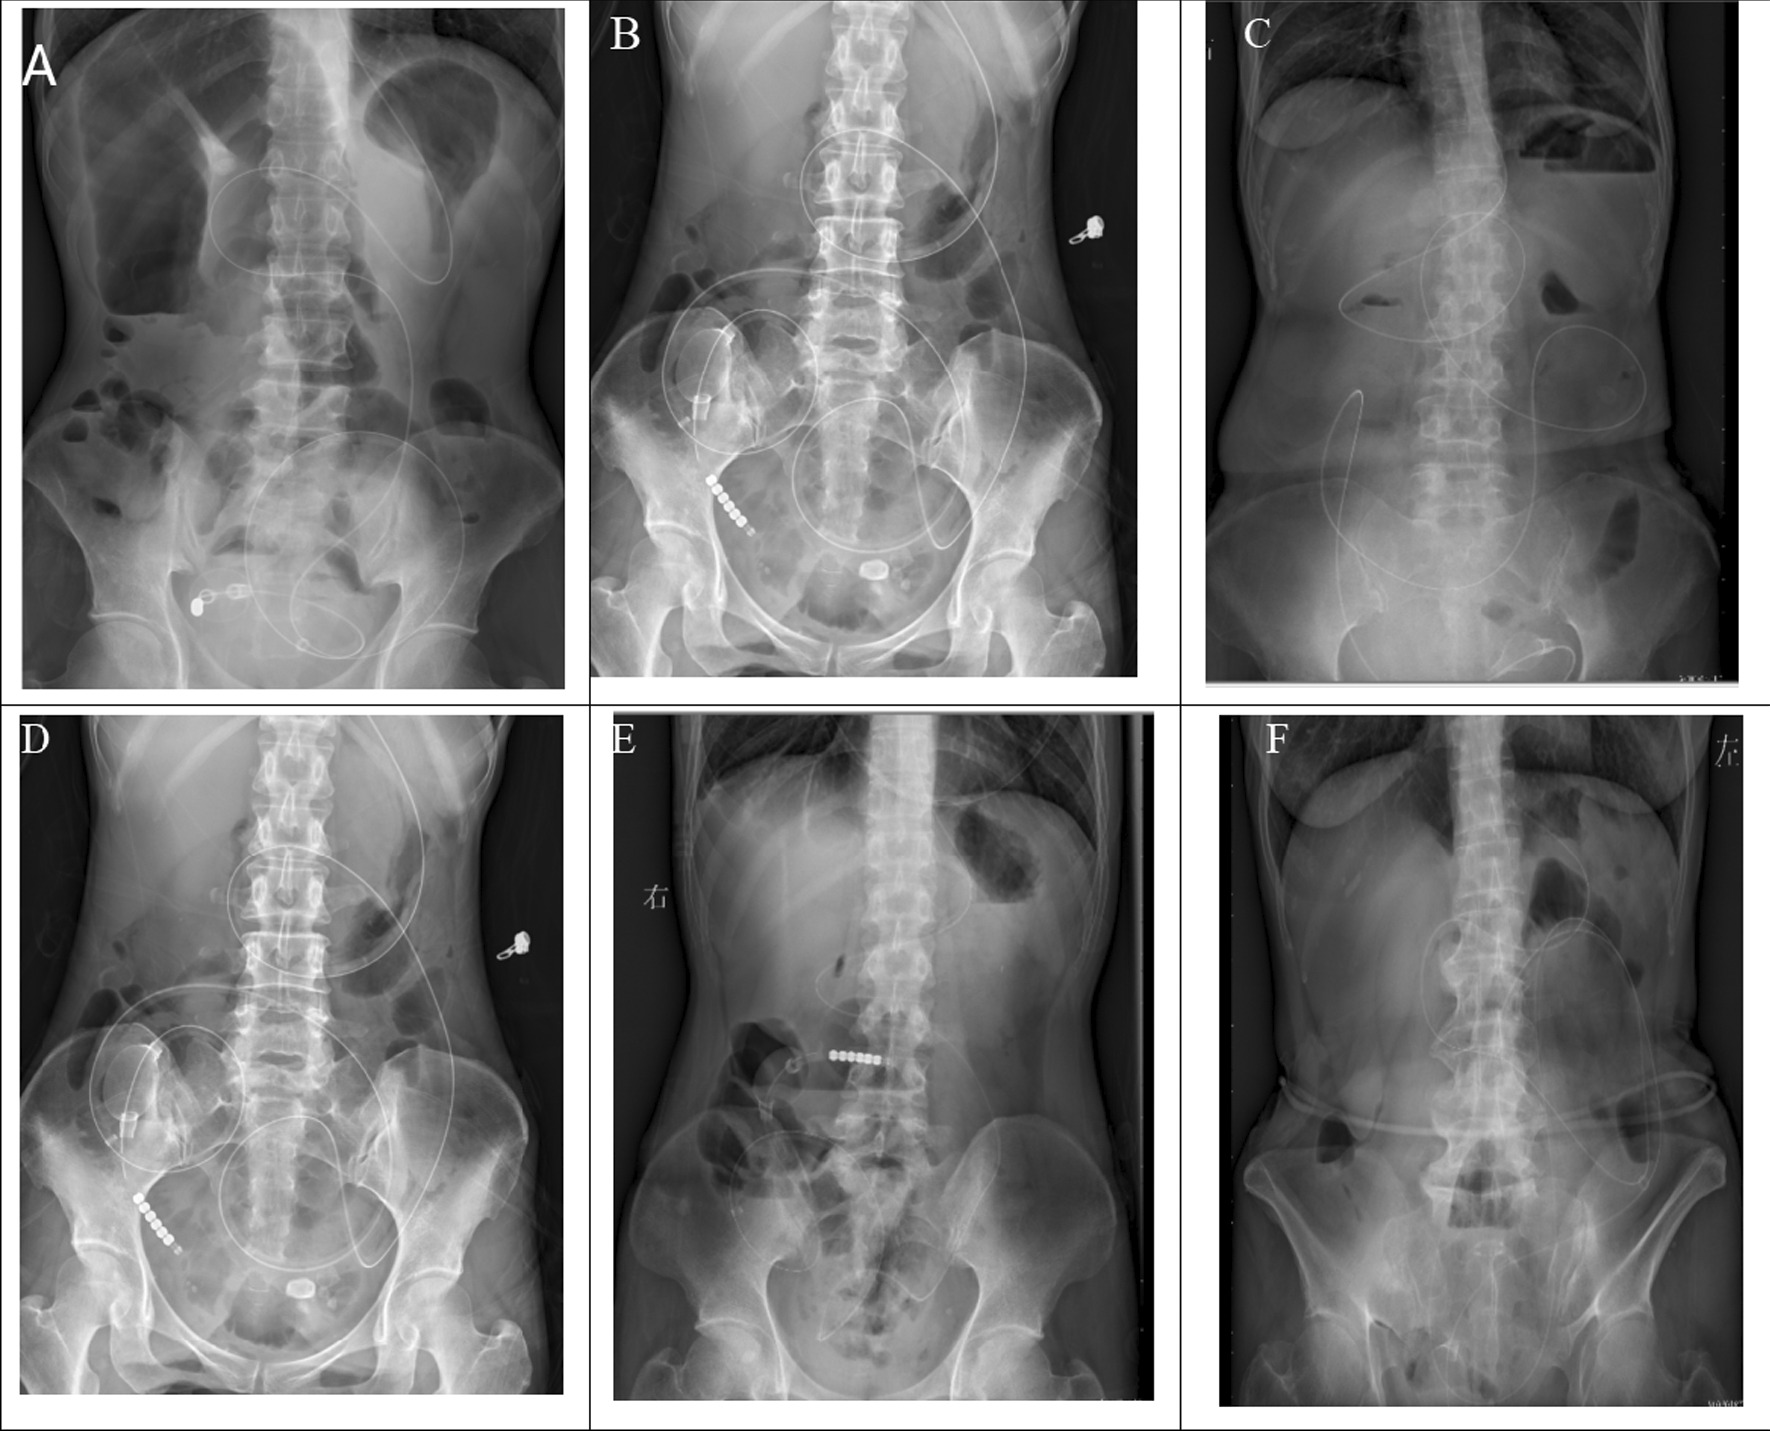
Relief after 2w-4w transnasal ileum tube insertion by X-ray. A image of X-RAY after transnasal ileum tube insertion of patient 1; B image of X-RAY after X-RAY of patient 2; C image of X-AY after transnasal ileum tube insertion of patient 3; D image of X-AY after transnasal ileum tube insertion of patient 4; E image of X-AY after transnasal ileum tube insertion of patient 5; F image of X-AY after transnasal ileum tube insertion of patient 6. B–F indicated fully relieved; A indicated partially relieved
